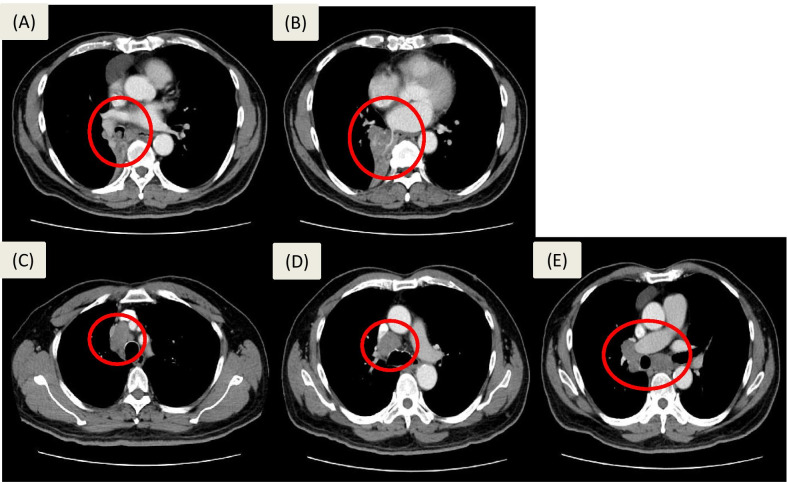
Series of chest CT scan images before treatment showing RLL lung tumor, cT4N2M0, stage IIIB, invading into the RIMB and right inferior pulmonary vein (a, b). Enlarged lymph nodes were noted at right para-tracheal, pre-carina, right hilar and sub-carina regions, compatible with metastatic lymphadenopathy (c, d, e). A cystic anterior mediastinal lesion was also noted
